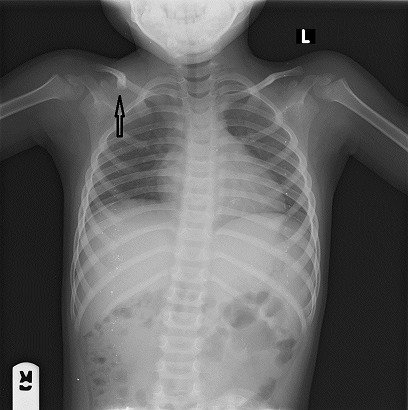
Chest radiography showing right clavicular fracture (black arrow)
